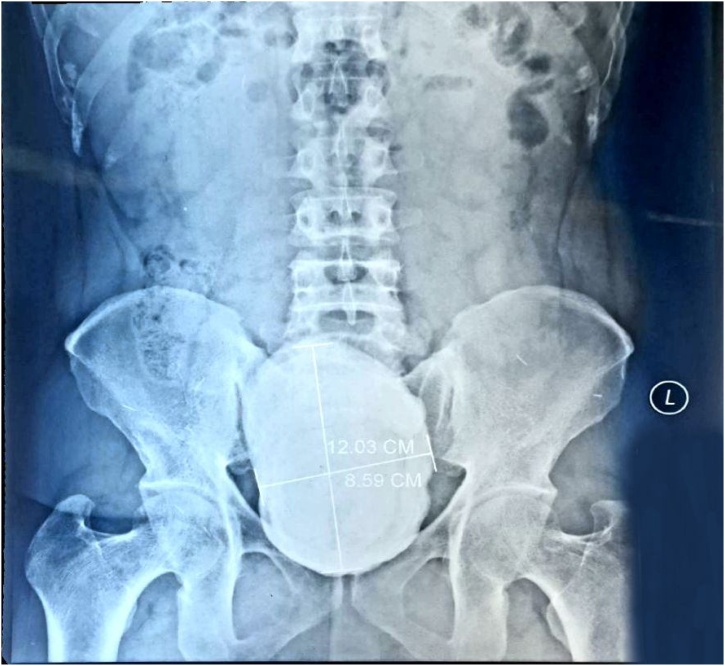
The patient’s BOF radiography shows a radiopaque round shape that occupies the bladder.
